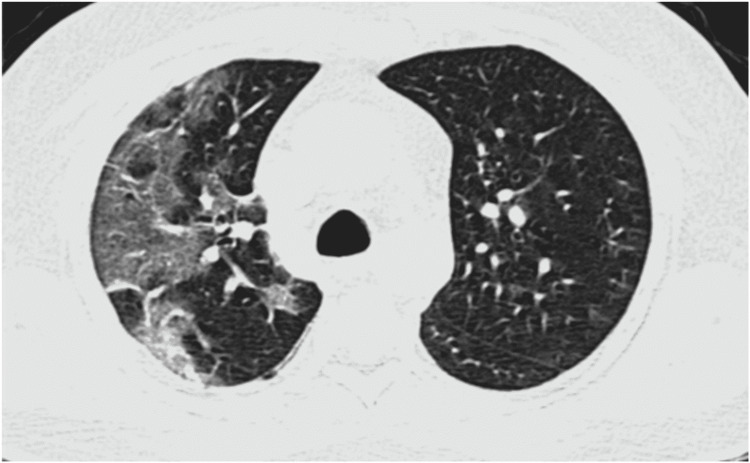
Axial CT section of the chest showing ground-glass opacities in a patient with COVID-19 pneumonia
